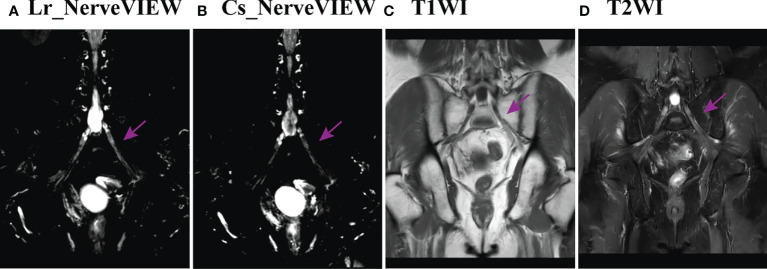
Example of the LSP structure in Lr_NerveVIEW (A), cs_NerveVIEW (B), T1-weighted (C) sequences, and T2-weighted fat-suppressing sequence (D) in the coronal view from patient #7. The purple arrow indicated the left S1 nerve root. The S1 nerve root was clearly seen on Lr_NerveVIEW and Cs_NerveVIEW sequences but appeared slightly “brighter” on the Lr_NerveVIEW sequence. Meanwhile, the same nerve root on T1WI and T2WI sequences appeared vaguer and had worse differentiation with surrounding tissues.
